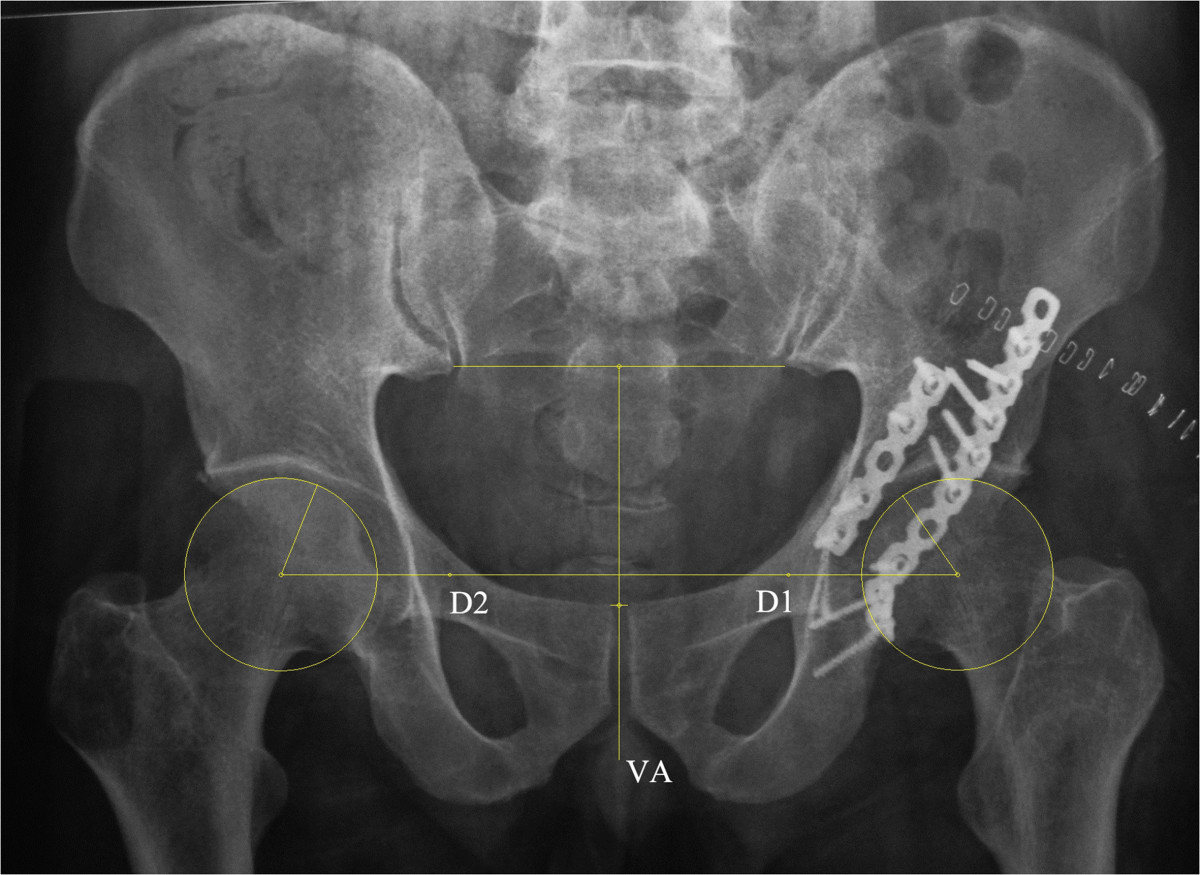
Radiographic measurement of the postoperative shift of hip joint center (HJC). The vertical axis of the pelvis (VA line) was defined by connecting the middle of the inter-sacroiliac line and the middle of the pubic symphysis. The distance (D1) between the postoperative femoral head center and the VA line was measured, the same as the distance (D2) between the contralateral intact femoral head center and the VA line. The horizontal shift (X) of the postoperative HJC was calculated as the absolute value of the difference between D1 and D2 (X = |D1 – D2|). The vertical shift (Y) was measured as the distance between the paralleled D1 line and D2 line.
